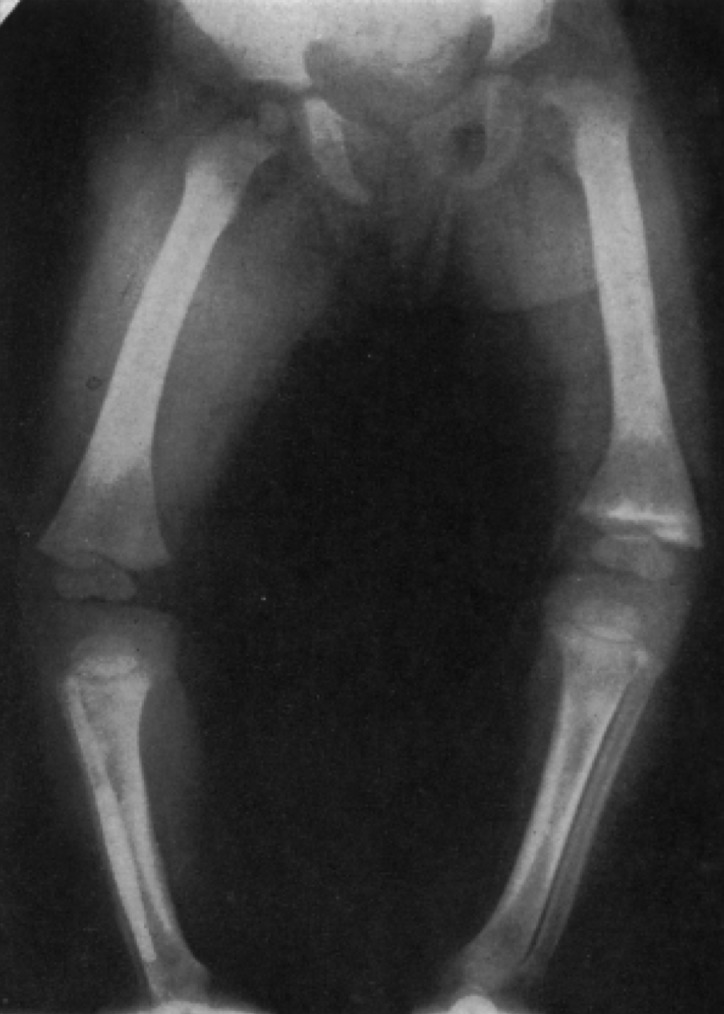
X-ray showing partial separation of a left upper femoral epiphysis.
